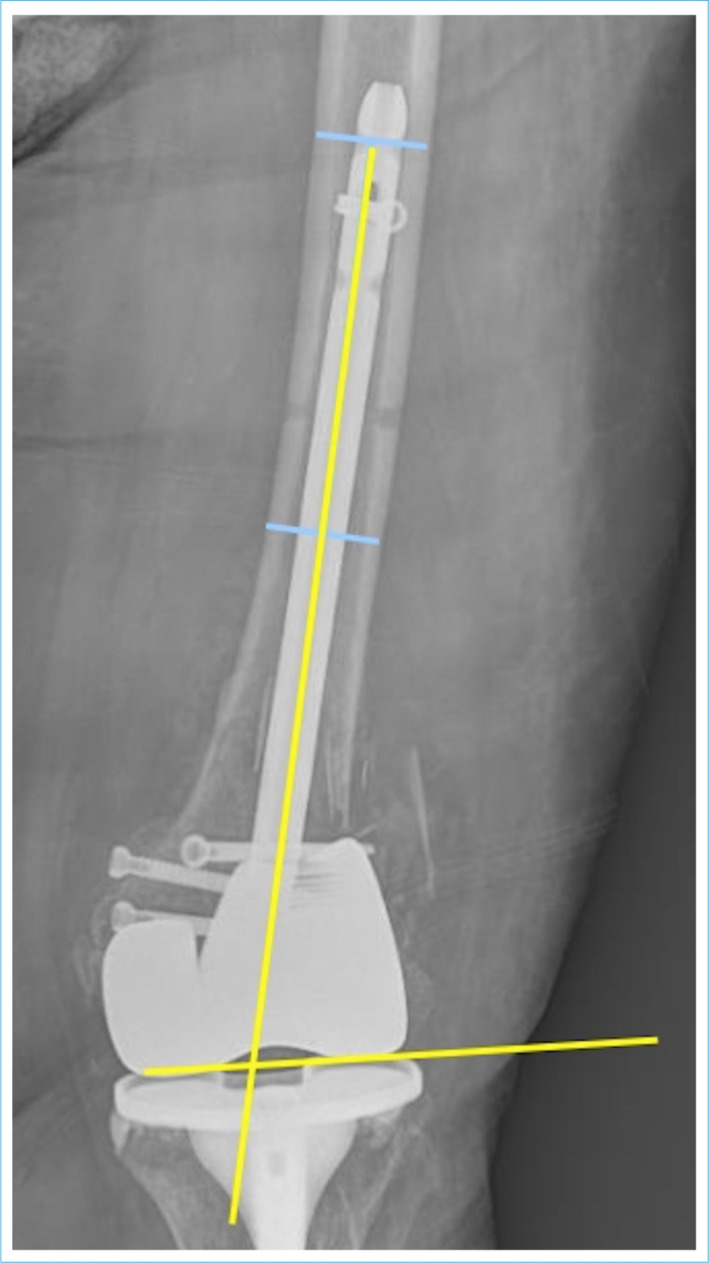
A – p X-ray of 72 year aged male patient with rIMN, 14.mos postoperatively. The alpha angle: 101°.
